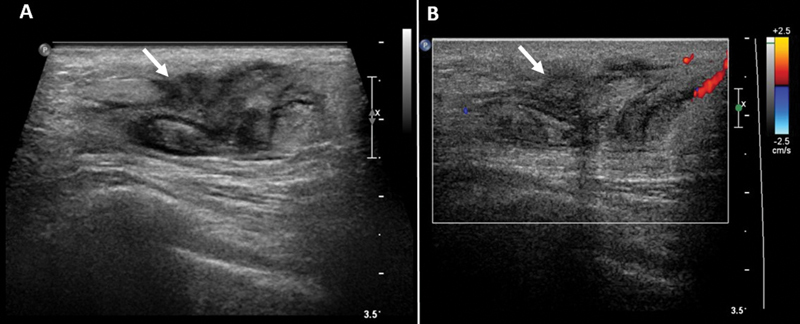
Left breast ultrasound (
